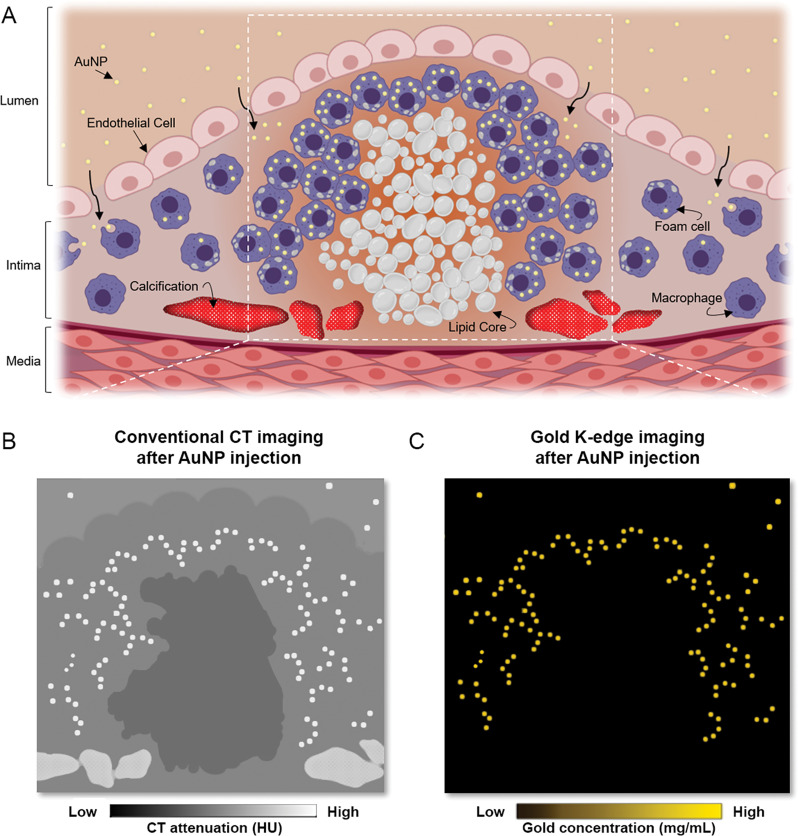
Molecular k-edge imaging approach using photon-counting CT in vivo for
